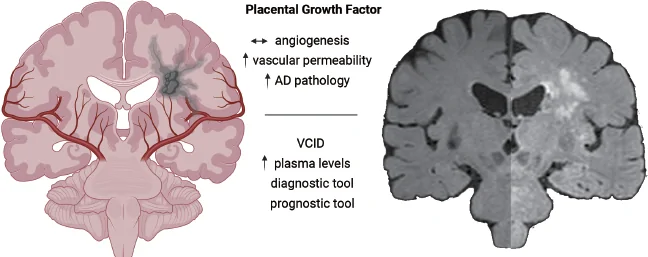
Placental growth factor regulates cerebral microvascular permeability and drives white matter lesions in vascular cognitive impairment and dementia (VCID). Normal levels of placental growth factor (PlGF) are necessary for brain vascular angiogenesis and the regulation of brain vascular permeability. When abnormal, PlGF increases brain vascular permeability and may synergize with Alzheimer’s disease (AD) pathologies. Elevated plasma levels of PlGF serve as diagnostic and prognostic biomarkers of vascular cognitive impairment and dementia associated with white matter hyperintensities on magnetic resonance imaging (MRI) (right) and can differentiate from normal MRI patterns (left). Created with BioRender.com.
